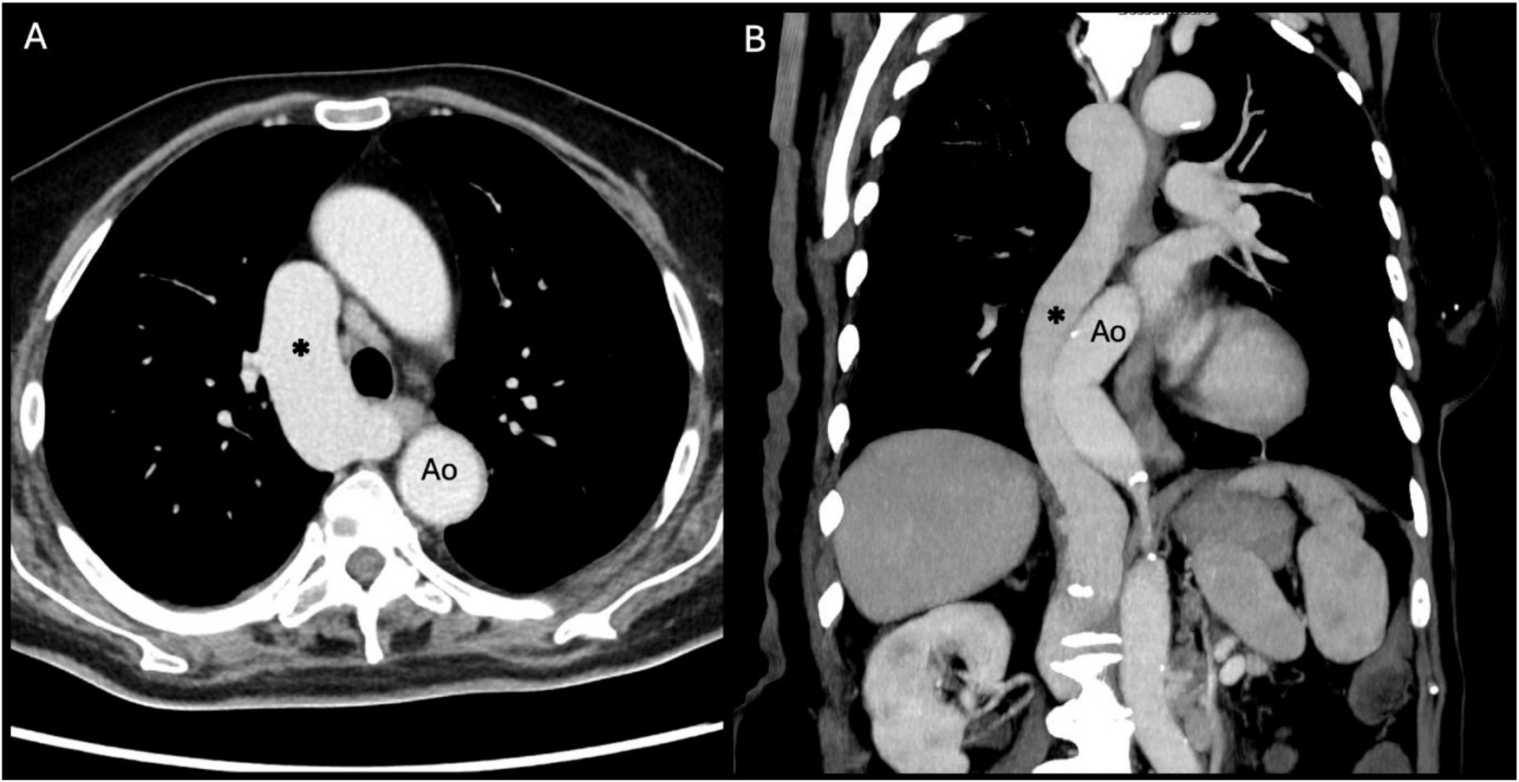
Contrast-enhanced computed tomography (CT) in mediastinal window, showing the thorax in axial (A) and coronal (B) views. A dilated vein is visible running to the right of the aorta (Ao), corresponding to the azygos continuation of the inferior vena cava (single black asterisk). Note in panel B, the presence of polysplenia.
